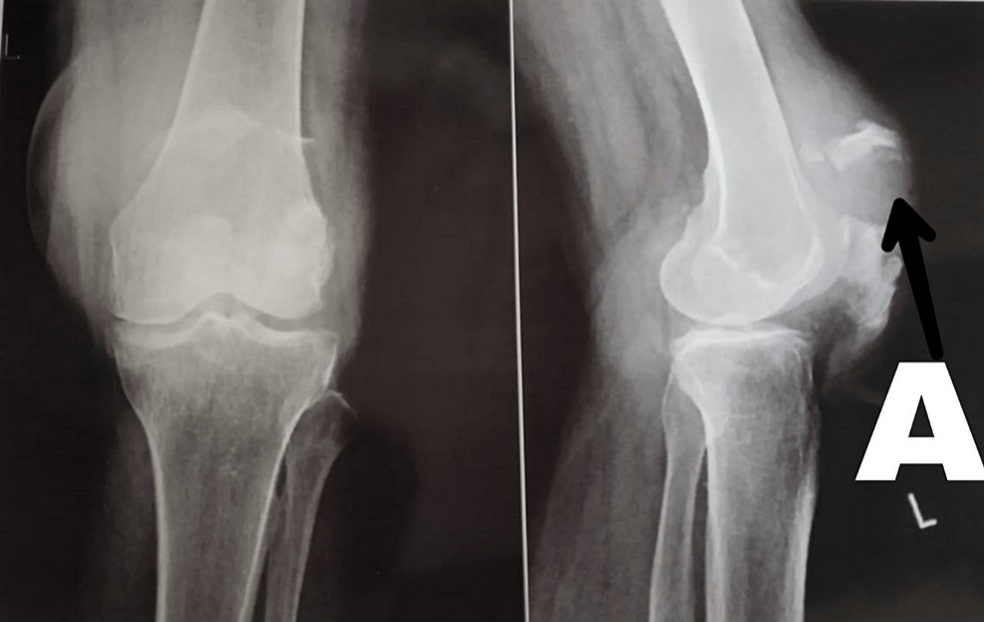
Preoperative X-ray of comminuted patella fracture
